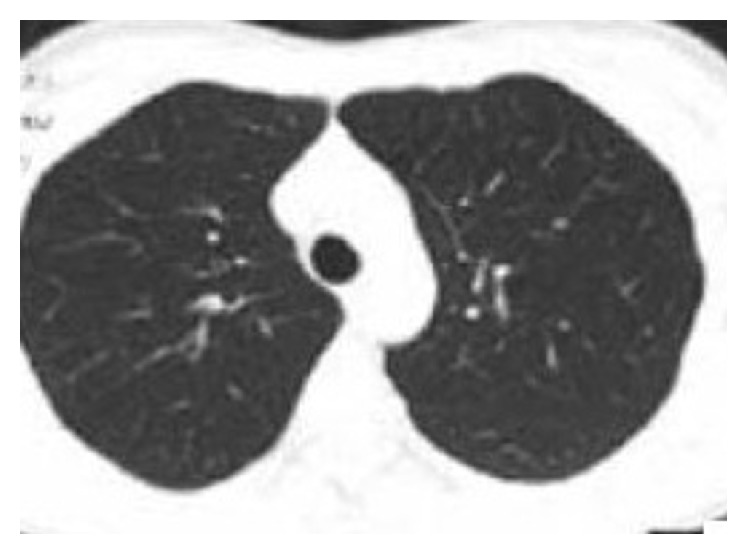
A normal HRCT chest obtained 2 months after discharge.
